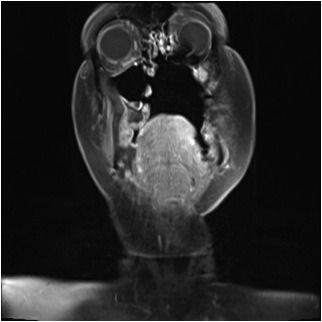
Coronal CT imaging following radical surgery to remove tumour.
